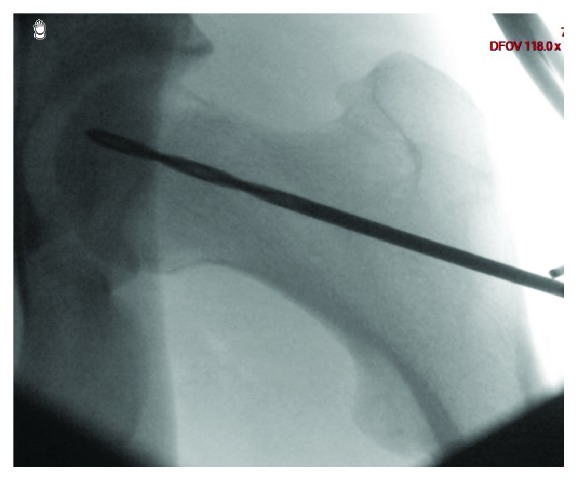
Left hip preoperative X-ray AP view.
